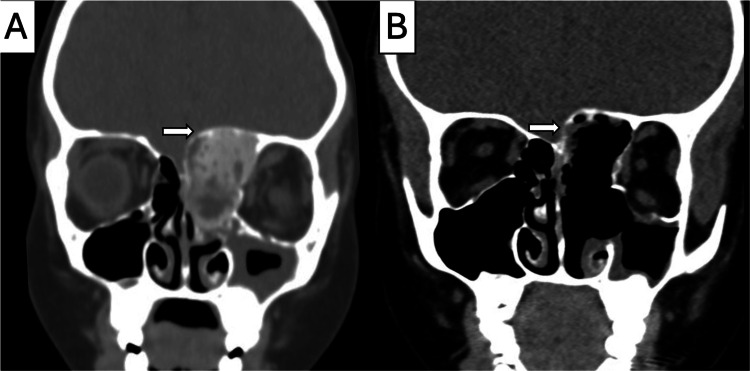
Case 1: Preoperative and postoperative CT. (A) Coronal view: a left ethmoidal tumour, heterogeneous with a central hypodense soft-tissue density, showing erosion of the lamina papyracea and anterior cranial base (white arrow). (B) Coronal view: post-surgical changes following maxillary antrostomy and left anterior ethmoidectomy; small remnants at the skull base were left in situ to avoid iatrogenic injury (white arrow).
